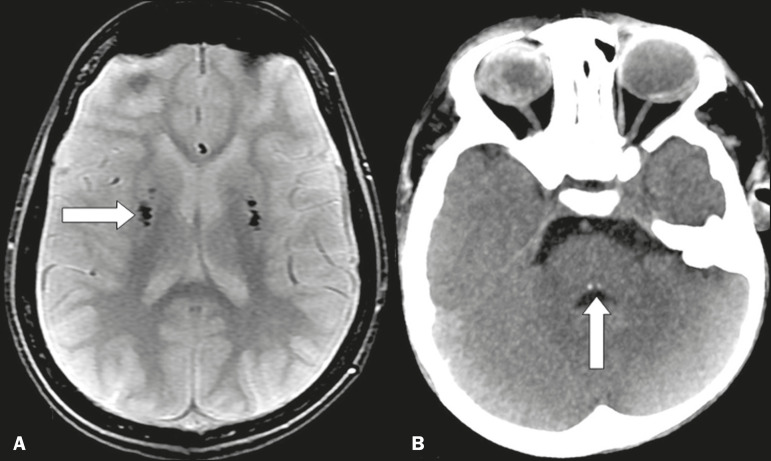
A: Axial T2*-weighted gradient-recalled echo MRI sequence of the skull, showing areas of markedly hypointense signals, corresponding to calcifications in the area of the lentiformis nuclei (arrow) in a case of Cockayne syndrome. B: Unenhanced axial cranial CT scan, with soft-tissue window settings, showing small calcifications in the posterior aspect of the brainstem, in the region of the abducens nuclei (arrow), in a case of Moebius syndrome.
